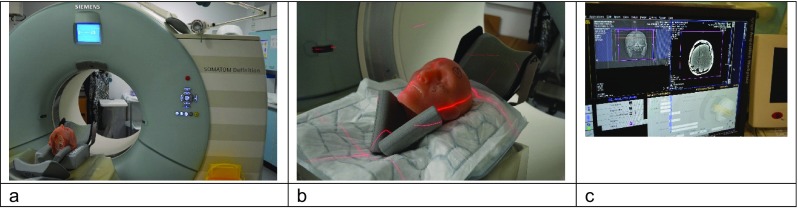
a Face 1/Golf in the CT scanner. (b) Detail of Face 5 /Kilo in the CT scanner. (c) CT work station displaying images from Face 2/Hotel; bullet tract and fractures are visible in the right-hand image on the screen
